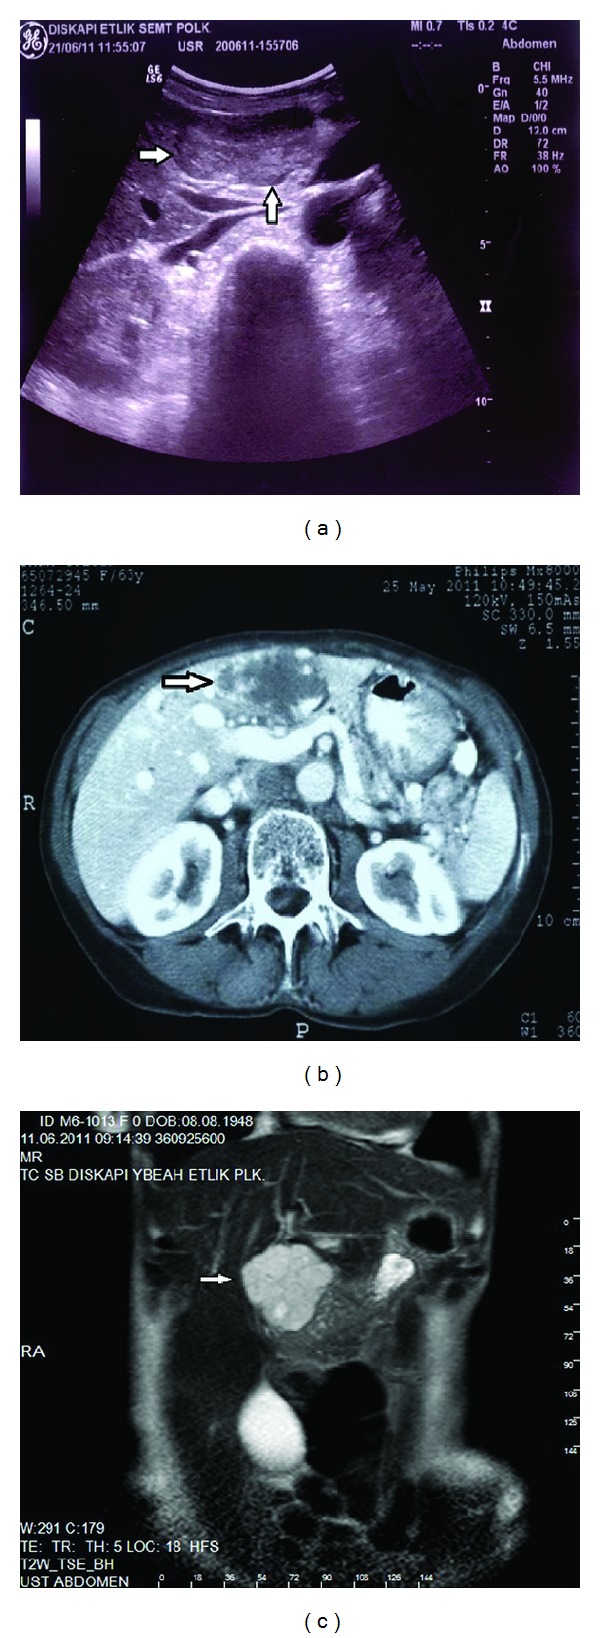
(a) A lobulated and countered, iso-hiperechogen liver mass in the left lobe on US imaging (arrows), (b) on postcontrast CT scan, nodular enhancement was seen at the periphery of the lesion (arrow). (c) On coronal T2W images, the lesion was hyperintense (arrow).
